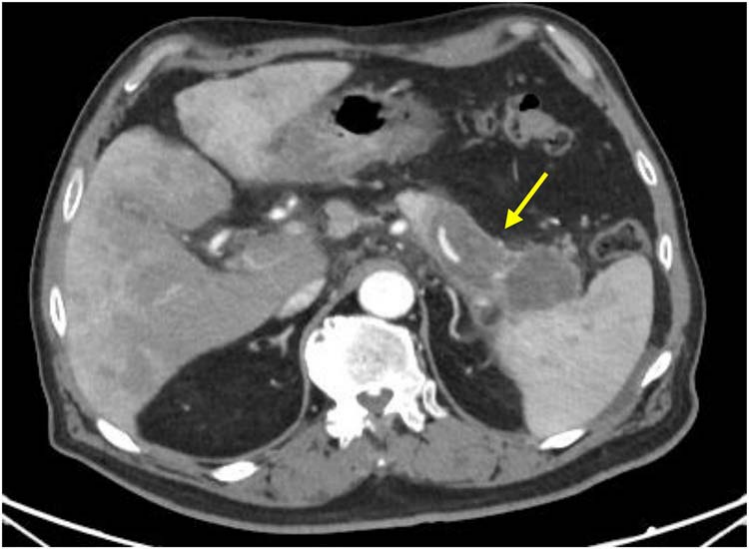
CT scan with IV contrast: axial image showing hypoenhancing infiltrative pancreatic tail mass with central necrosis (arrow), measuring 8 × 3 × 4 cm with adjacent stranding and mass abutting the spleen that appears to completely encase the splenic artery and vein.
